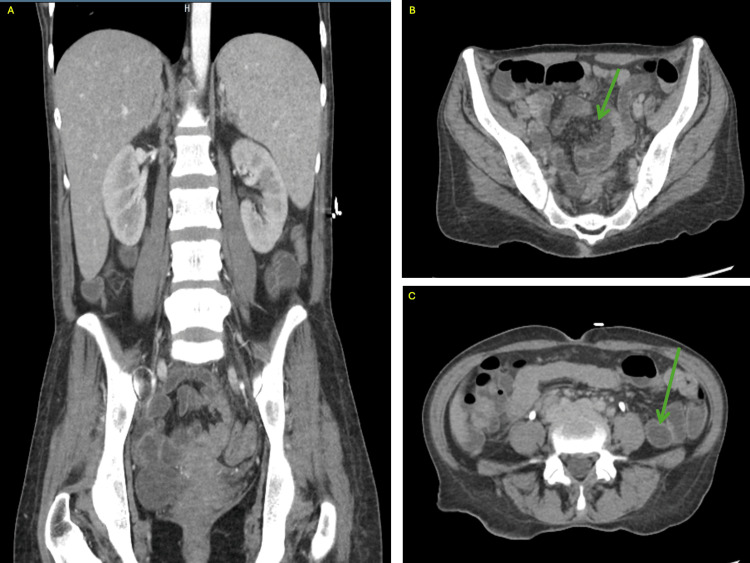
Computed tomography imaging of the abdomen and pelvis showing hepatosplenomegaly and non-specific enteritis A:Coronal view demonstrating substantial hepatosplenomegaly. B-C: Axial views demonstrating infectious versus inflammatory enteritis.
